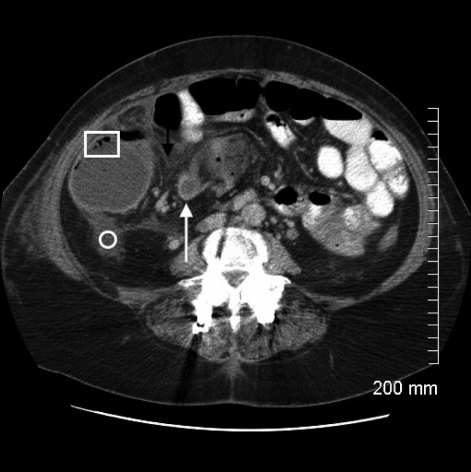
Axial CT image demonstrates twisting of the mesenteric vessels producing the ”CT whirl“ sign, which is highly specific for intestinal volvulus (white arrow). Again demonstrated are the associated pneumatosis intestinalis (square) and pericolonic free fluid (circle).
